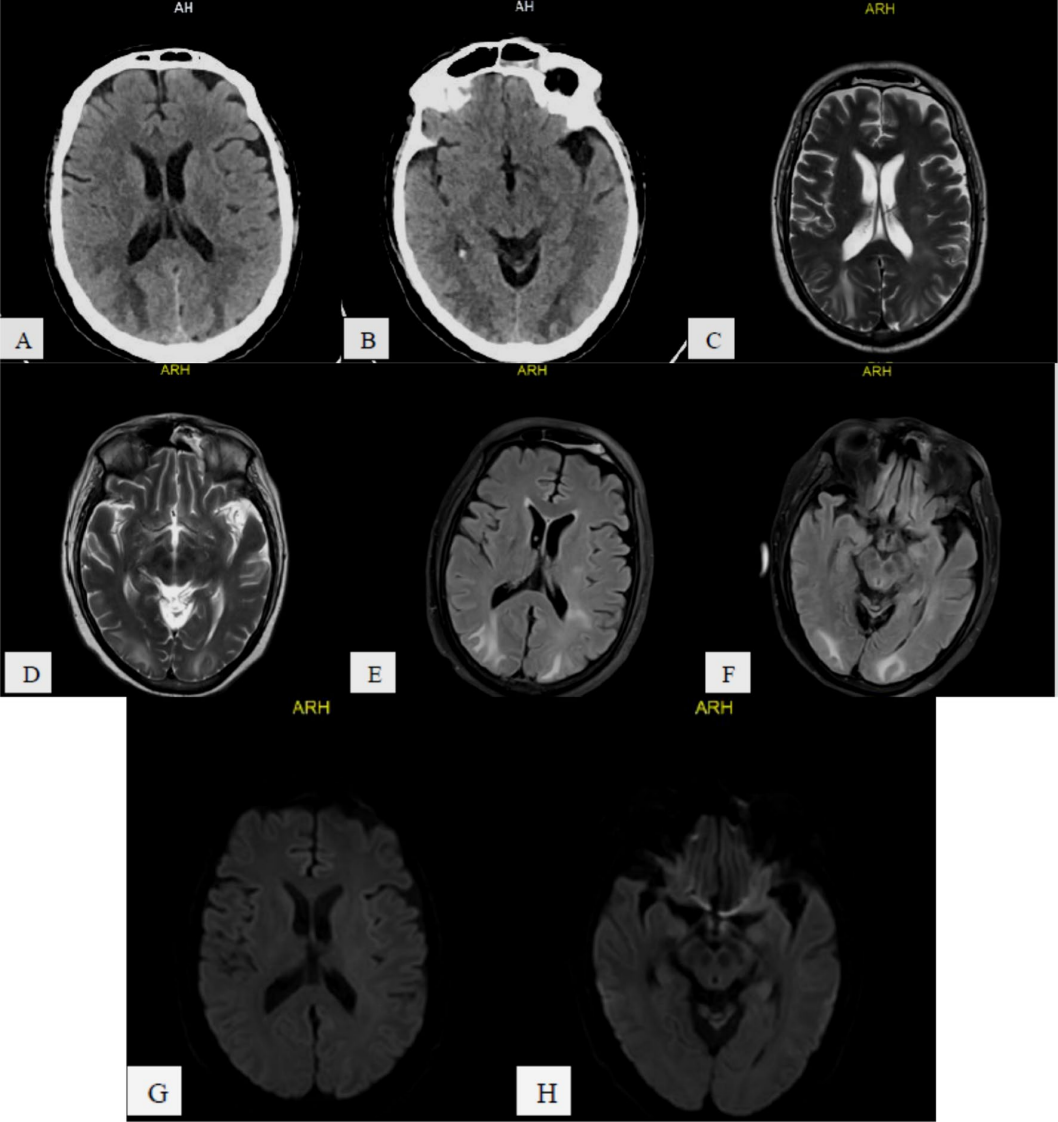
47-year-old COVID-19 male patient with first time GTC seizure after prolonged ICU admission A, B axial CT images, C, D T2 WI, E, F FLAIR, G, H DWI showing bilateral posterior parieto-occipital and to less extent high frontal subcortical and deep white matter hyperintense signal noted on T2 and FLAIR images with morphological vasogenic edema pattern. No significant mass effect. It shows free diffusion features are impressive of morphological *PRES* (*posterior*
